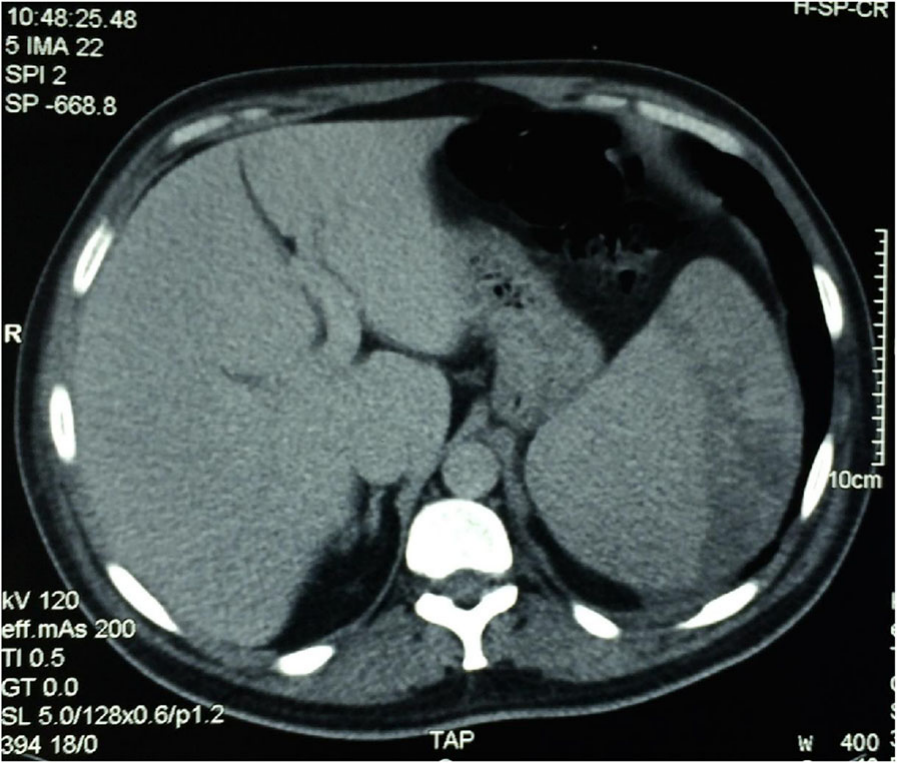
CT scan abdomen showing a 20 cm large spleen with multiple hypodensities (infarcts)
